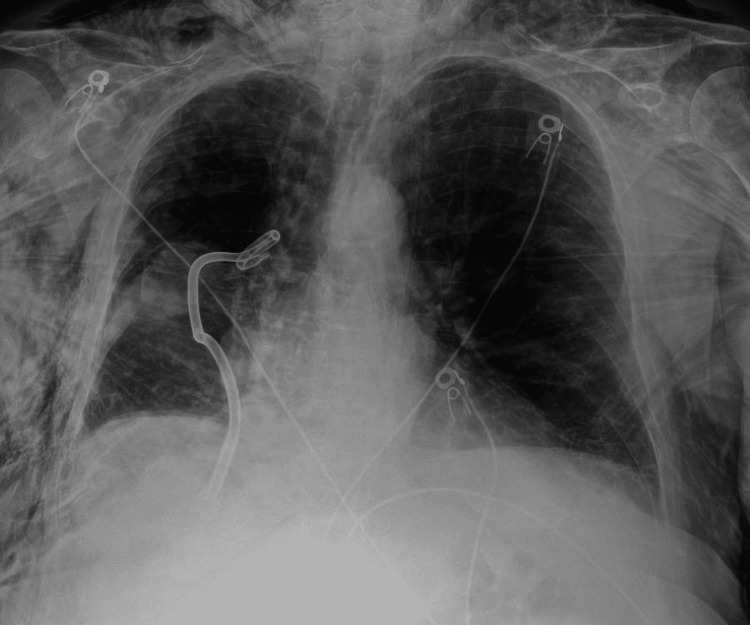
Chest radiograph showing persistent right pneumothorax despite placement of a 14-French pigtail catheter.
